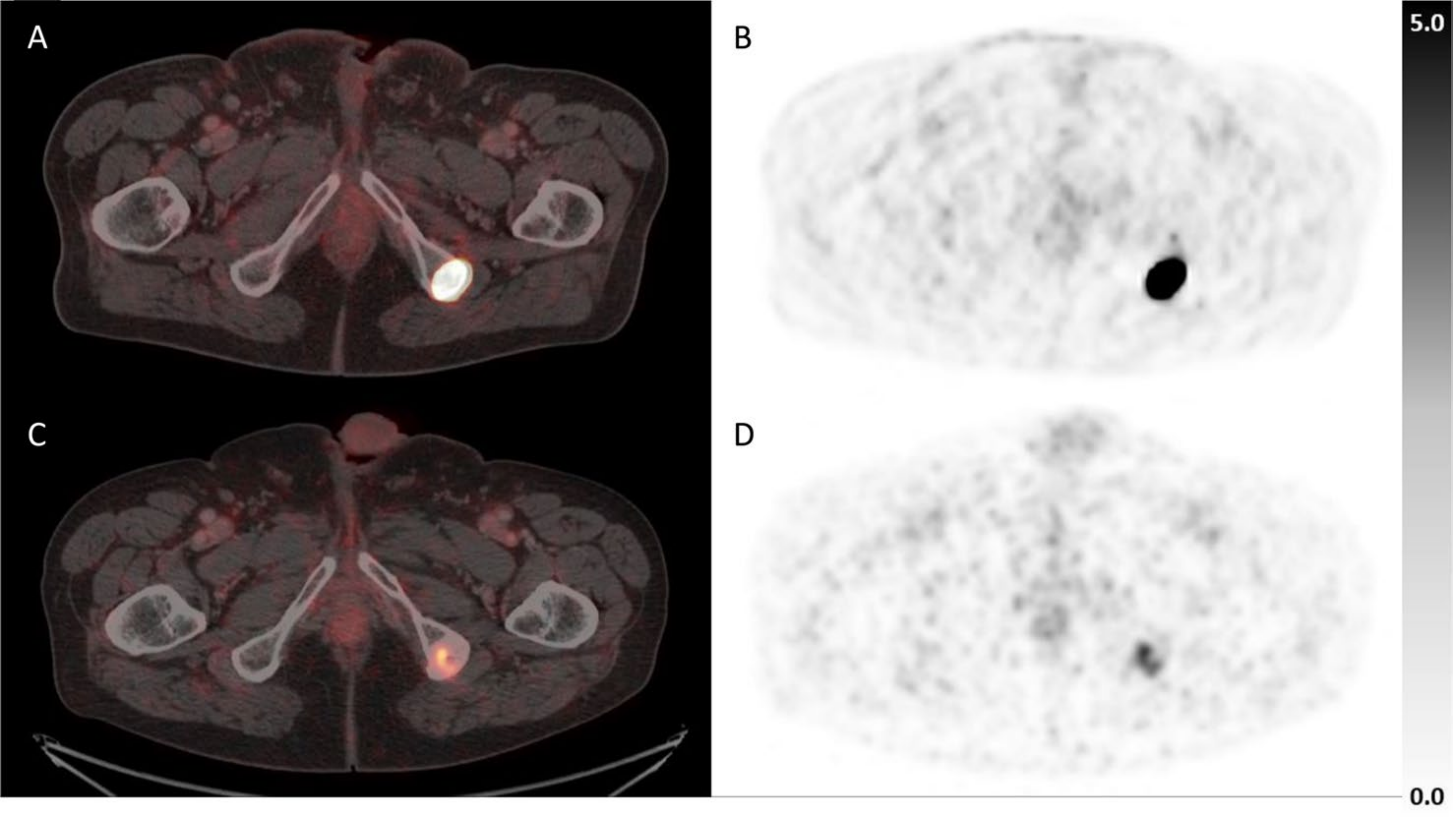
Example of bone metastasis with PR, fused PET/CT and PET only prior to (A + B) and after MDT (C + D)
